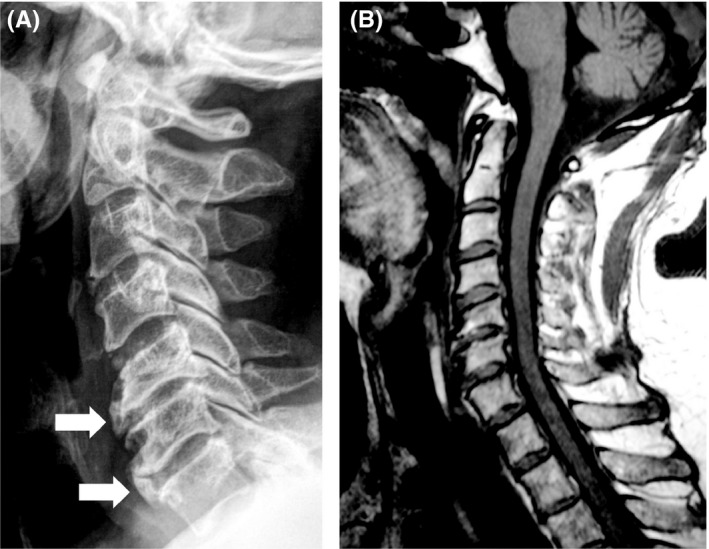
A, Plain radiograph of the cervical spine revealing a diffuse idiopathic skeletal hyperostosis with an ossification of the posterior longitudinal ligament associated to an anterior longitudinal ligament ossification (arrow). B, MRI of the cervical spine showed a compression on the adjacent wall of the esophagus. There is no spinal cord compression
